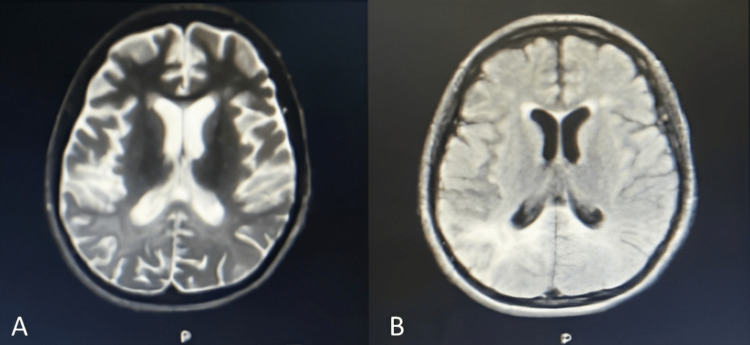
Cranial MRI as seen on axial view showing hyperintensities in the cerebral cortical, subcortical, and periventricular white matter changes on the bilateral parietooccipital and temporal areas on T2 weighted (A) and FLAIR (B) sequences. MRI: magnetic resonance imaging; FLAIR: fluid-attenuated inversion recovery
